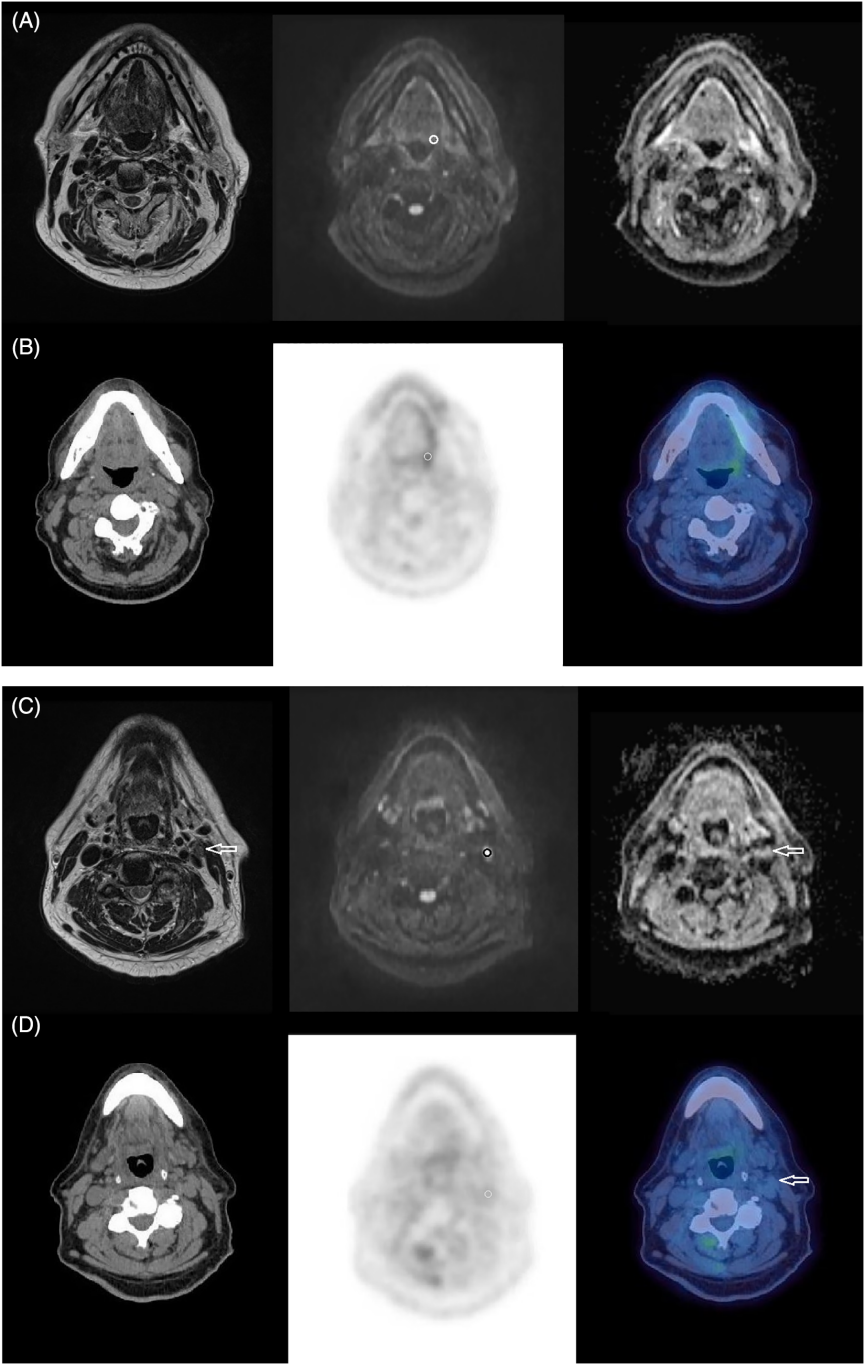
12‐week post‐chemo‐radiotherapy MRI and PET/CT study in the same patient. T2w, *b* = 800 DWI and ADC map (*b* = 100‐800) MRI images (A and C) and CT, PET, PET/CT fused image (B and D) indicating the site of the previous left glosso‐tonsillar sulcus tumor, which is now nonmeasurable and left level 2 lymph node, which has markedly reduced in size (arrows in C and D). The 6 mm ROIs on the MRI study are placed at the site of the previous primary tumor (circle in A) and at the residual nodal tumor as indicated (circle in B). The corresponding 6 mm VOI on the PET‐CT study is seen within the central portion of the primary and nodal tumor (circles in B and D)
